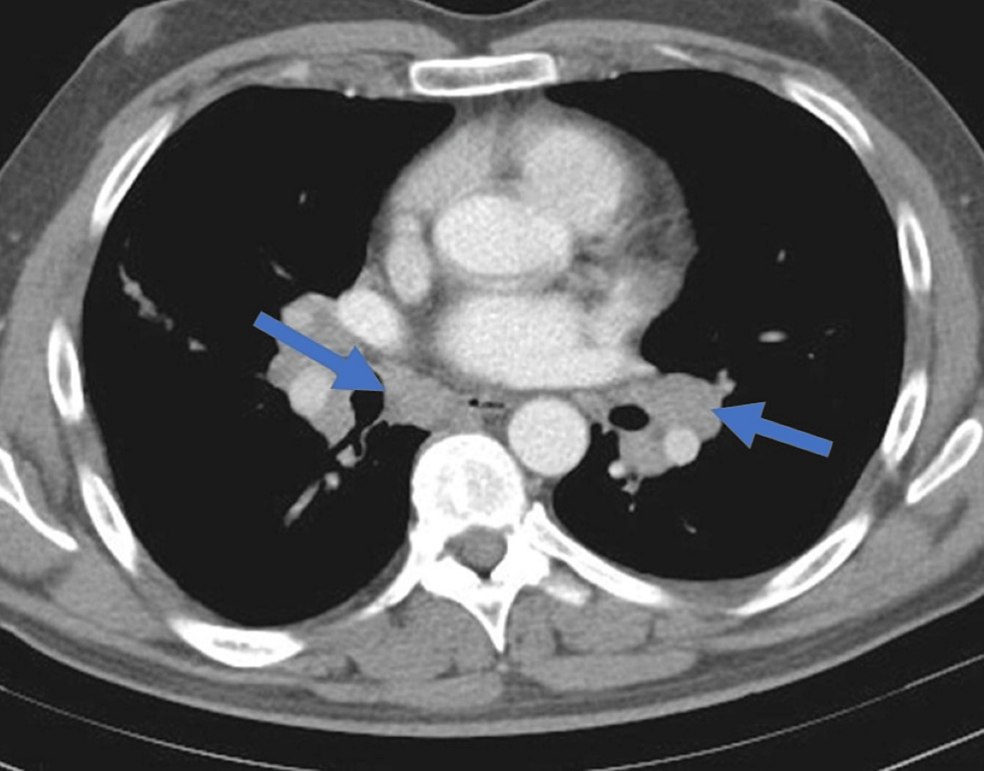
Chest computed tomography revealing bilateral hilar lymphadenopathy (blue arrows).
